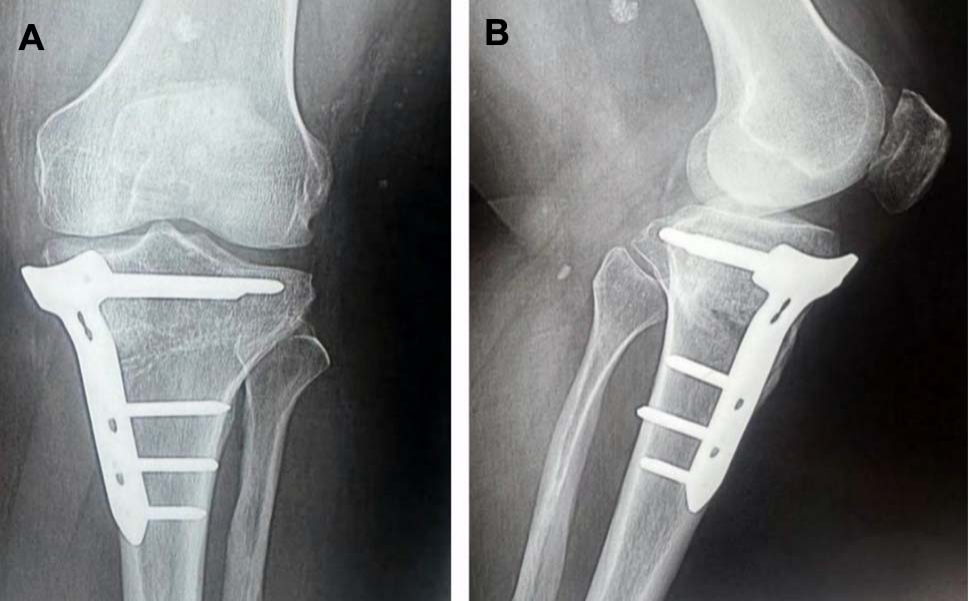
A 6-week postoperative knee X-ray (A) anteroposterior and (B) lateral views, showing healing of osteotomy site.
